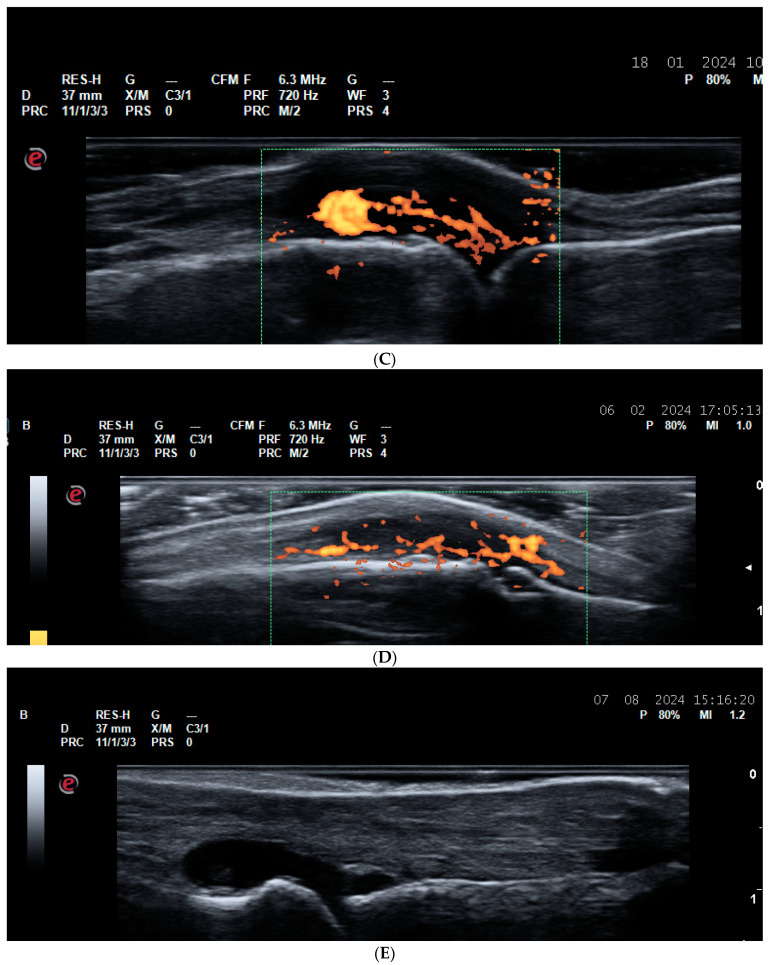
Synovitis. (A) A dorsal longitudinal scan of the wrist joint on Gray Scale Ultrasound (GSUS) in an RA patient—Grade 3 synovitis of the radiocarpal and intercarpal joints; (B) a dorsal longitudinal scan of the wrist joint on Power Doppler Ultrasound (PDUS) in an RA patient—Grade 2 synovitis; (C) a dorsal longitudinal scan of the second metacarpophalangeal (MCP) joint on PDUS in an RA patient—Grade 3 synovitis; (D) a dorsal longitudinal scan of the third proximal interphalangeal (PIP) joint on PDUS in an RA patient—Grade 3 synovitis; (E) a dorsal longitudinal scan of the first metatarsophalangeal (MTP) joint on GSUS—effusion and grade 3 synovitis.
